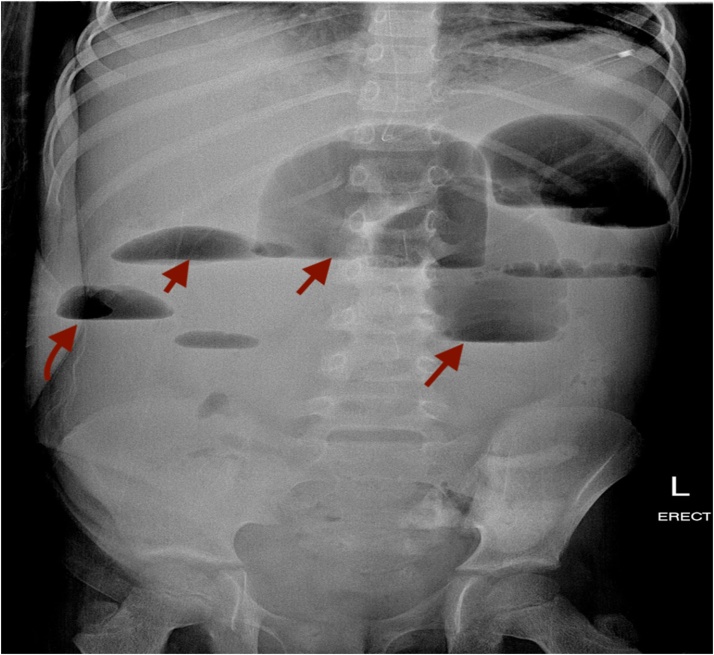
Post-operative abdominal X-ray showing multiple air fluid levels.
